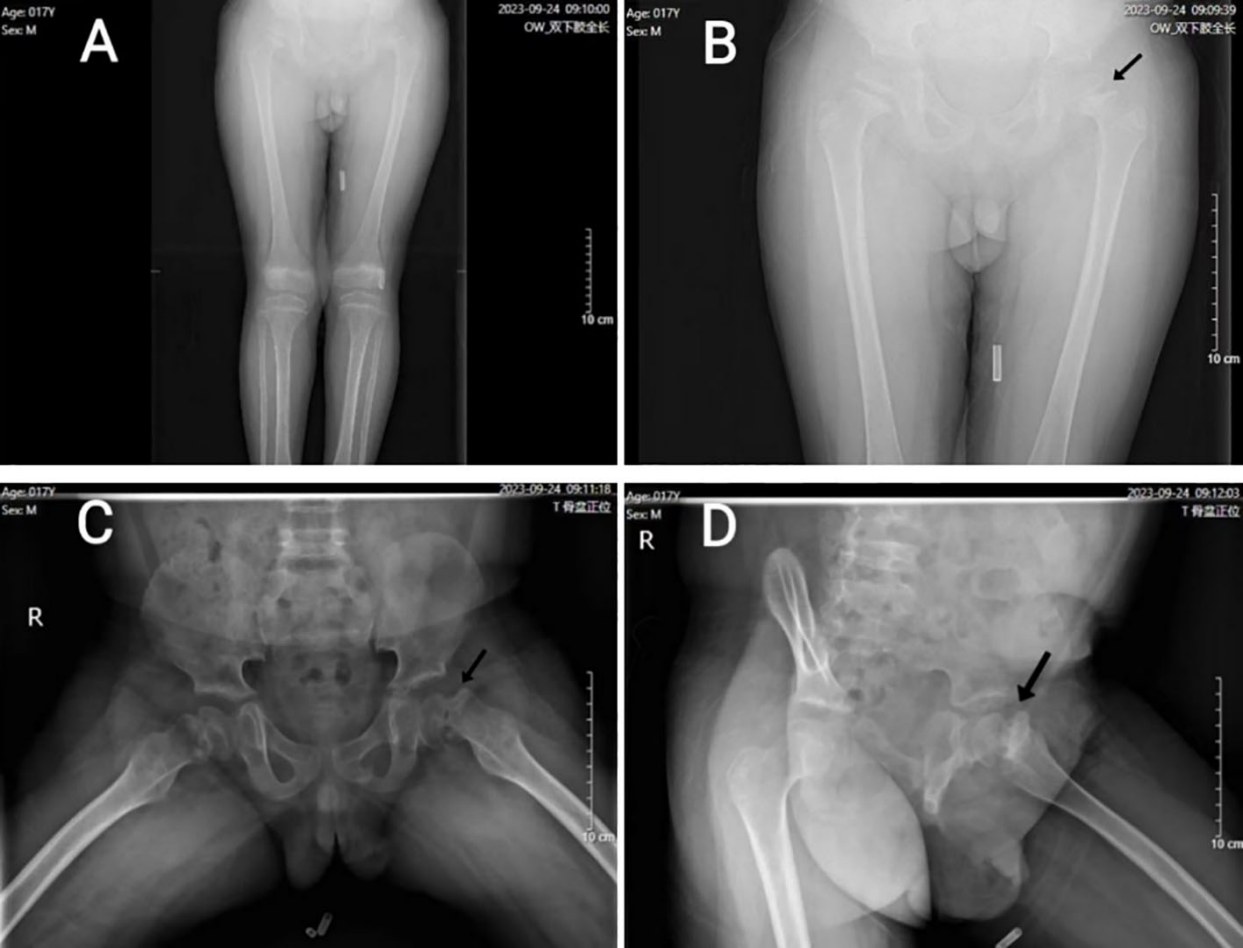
(A) (anteroposterior X-ray of both lower limbs): Both lower limbs were basically equal in length, and the long bones were lightly developed. (B) (hip and both upper limb’s anteroposterior X-ray of hip) and (C) (frog-like anteroposterior X-ray of hip): Bilateral femoral head epiphyseal plate widened, irregular edge; The joint surface of bilateral acetabulum was coarse, and the edge of bilateral ilium showed serrated changes. (D) (frog-like lateral X-ray of hip): the left femoral head was displaced medially, and the joint surface of each acetabulum was coarse; the left femoral epiphyses were separated (indicated by black arrows).
